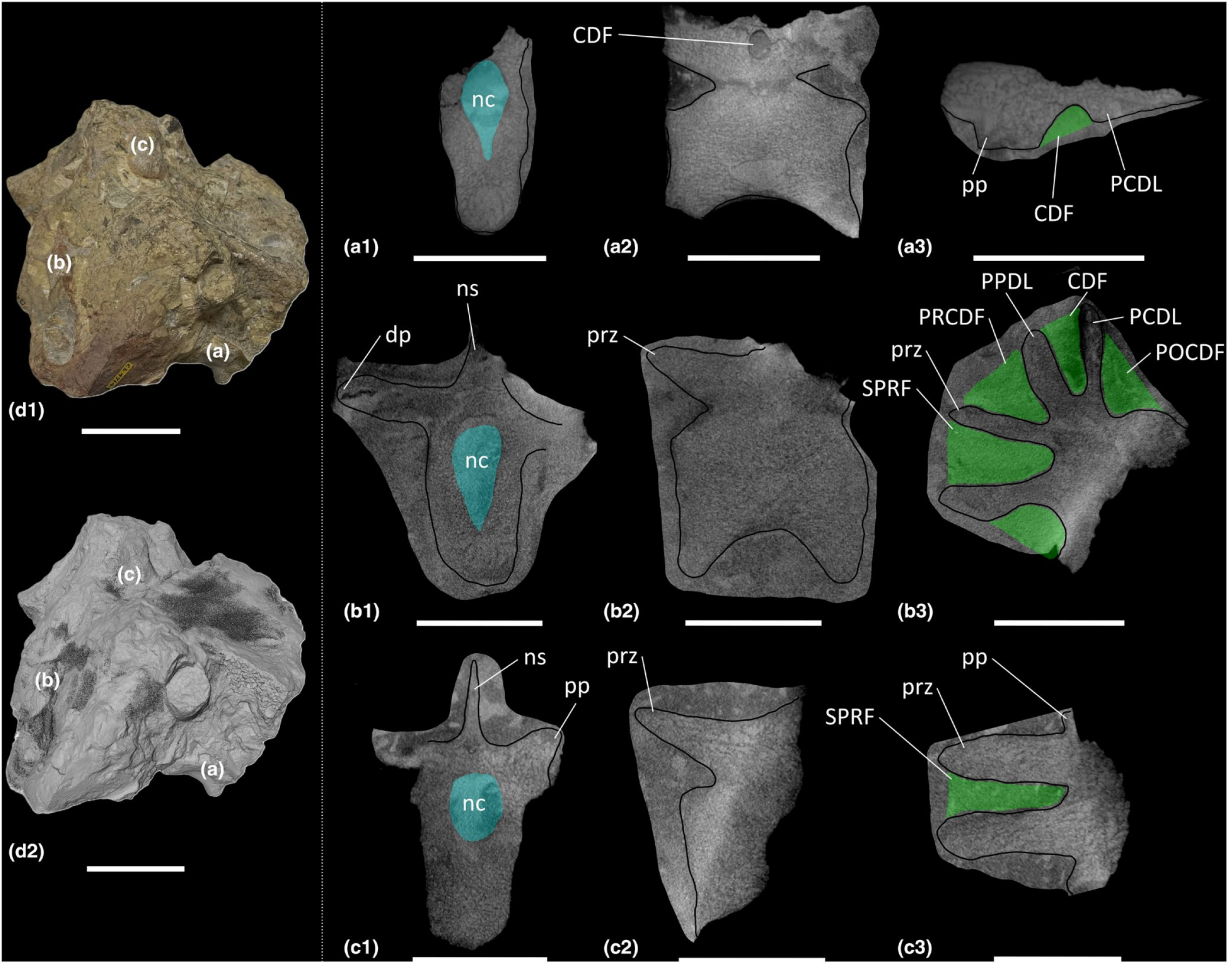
CT scan data of *Thecodontosaurus antiquus* dorsal vertebrae in Cb 4714 block. (a) element A, (b) element B, (c) element C; (a1–c1) transverse cross‐section, (a2–c2) parasagittal cross‐section, (a3–c3) horizontal cross‐section; (d1) photograph of block, (d2) surface scan of block. CDF, centrodiapophyseal fossa; dp, diapophysis; nc, neural canal; ns, neural spine; PCDL, posterior centrodiapophyseal lamina; POCDF, postzygapophyseal centrodiapophyseal fossa; pp., parapophysis; PPDL, parapodiapophyseal lamina; PRCDF, prezygapophyseal centrodiapophyseal fossa; prz, prezygapophysis; SPRF, spinoprezygapophyseal fossa. Key: Blue highlight, neural canal; green highlight, fossa. Scale bars = 2 cm (a–c), 5 cm (d).
